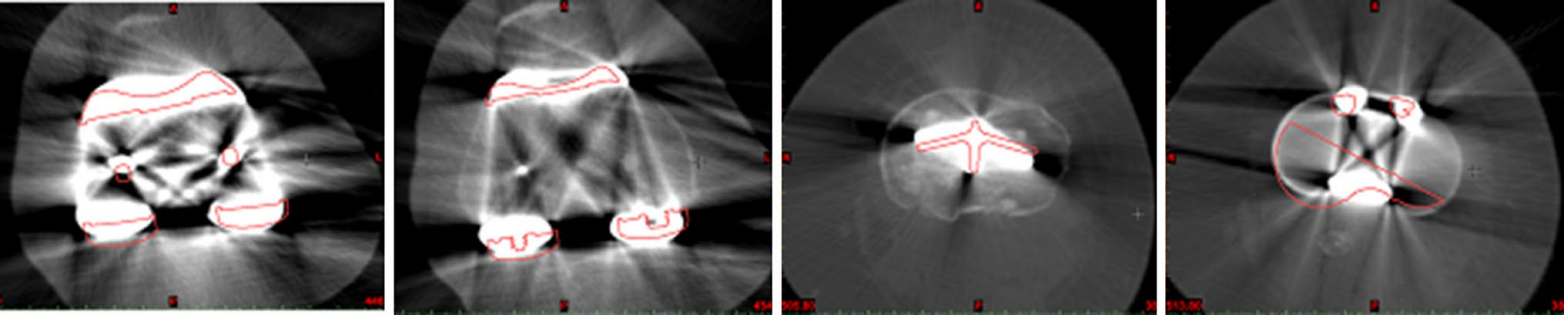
Example of the post-op CT scan images of femur (*first two images*) and tibia (*last two images*) with pre-op plan superimposed (*red*). This registration permits measurement of rotational alignment in the transverse plane
